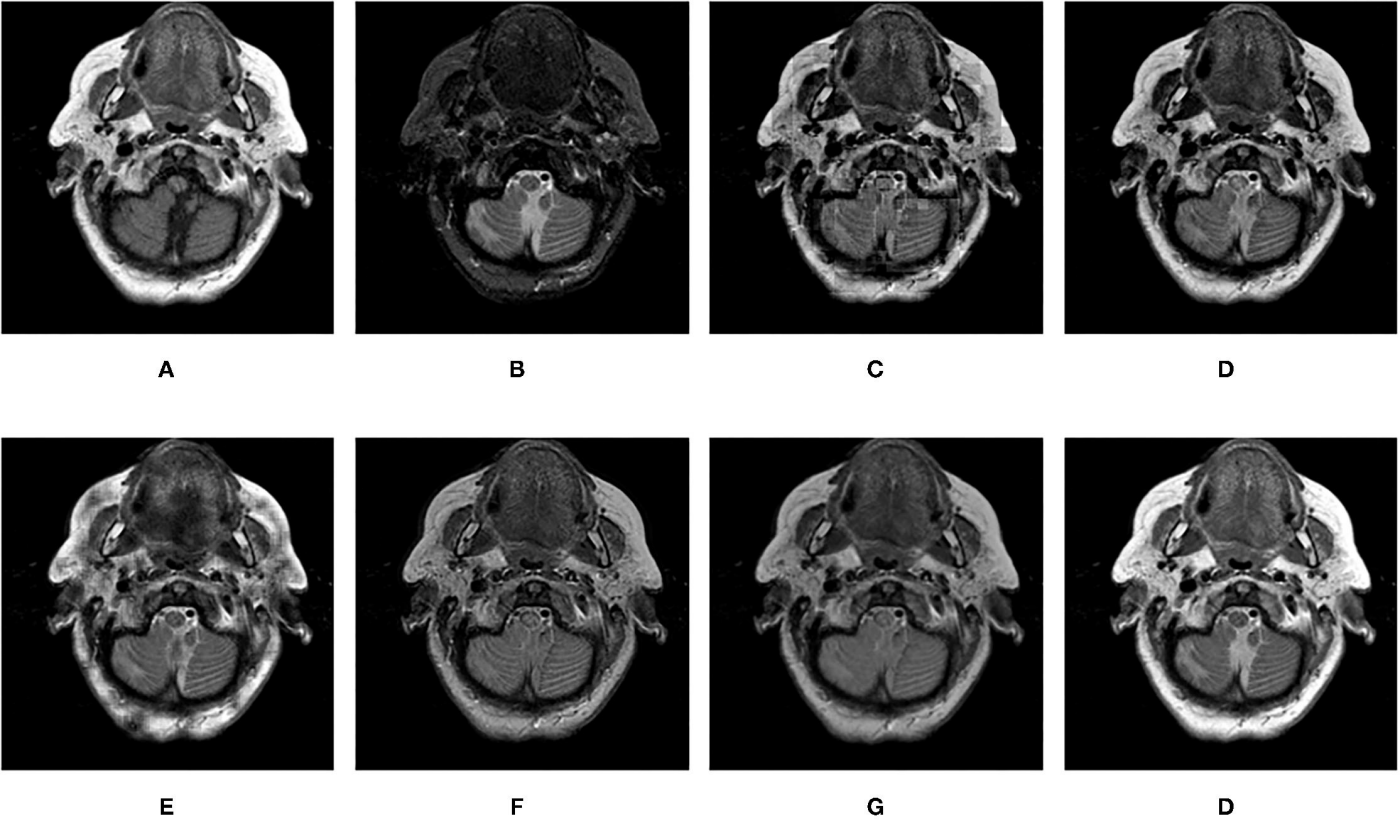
Comparison experimental results of CT and MRI images. (A) original image (CT); (B) original image (MRI); (C) DWT; (D) LP; (E) SR; (F) DTCWT-SR; (G) VGG; (H) MPS.
